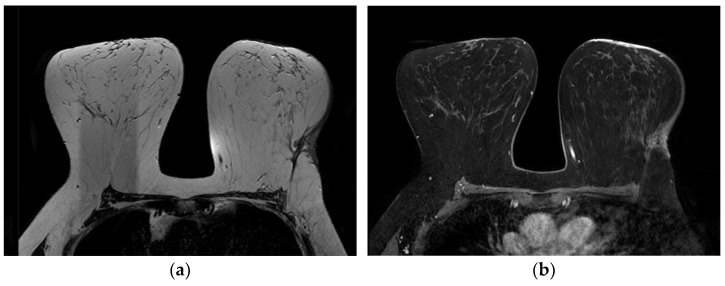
A 58-year-old patient with a history of carcinoma of the left breast treated with quadrantectomy and radiotherapy. (a) T2-weighted and (b) 3D gradient echo T1-weighted post-contrast images show skin thickening near the scar and late contrast enhancement. Punch biopsy revealed SBA.
